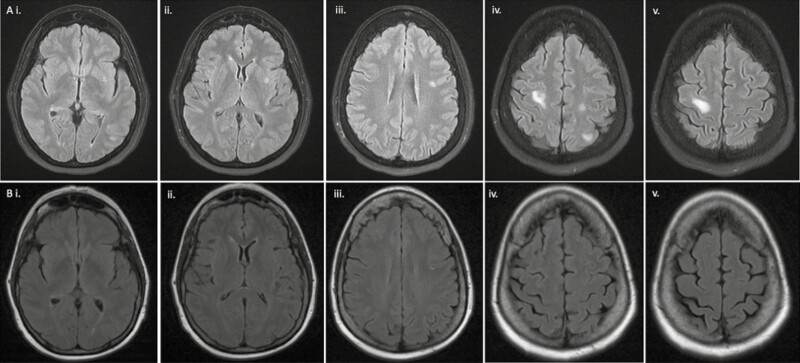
Comparison of T2 fluid-attenuated inversion recovery (FLAIR) MRI of brain metastases before (A) and after (B) tamoxifen therapy. (A): T2 FLAIR MRI on 10/25/2019, pre-tamoxifen therapy (i–v). (B): T2 FLAIR MRI on 2/10/2020, post-tamoxifen therapy (i–v).
